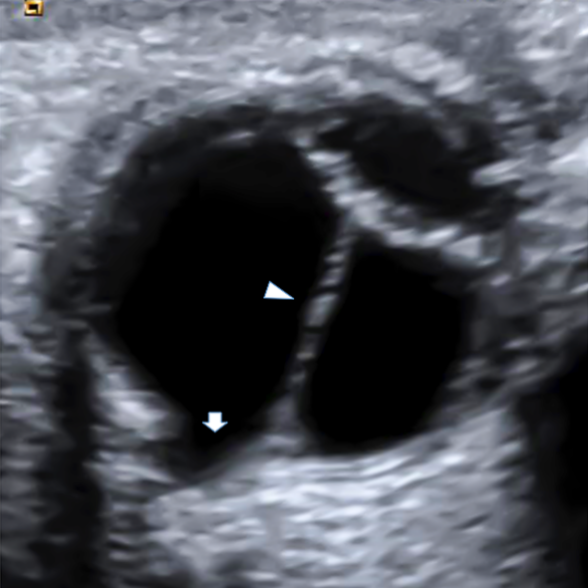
Cataract, a retrolental vascularized mass extending from the optic disc to the posterior lens capsule, and depression and enlargement of the optic disc in the right eye by Doppler ultrasonography
